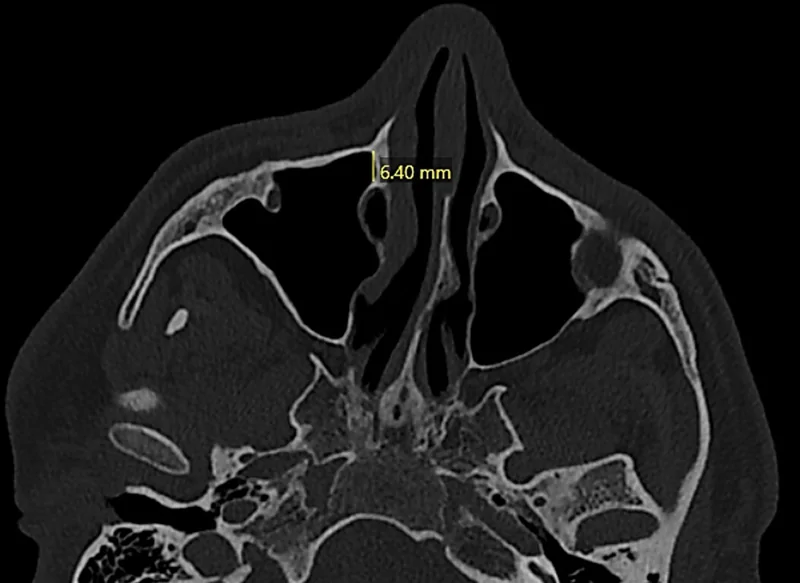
Computed tomography scan showing medium grade feasibility of the TPRA in right healthy sinus type IIb, with the yellow line representing the distance of the TPRA.
